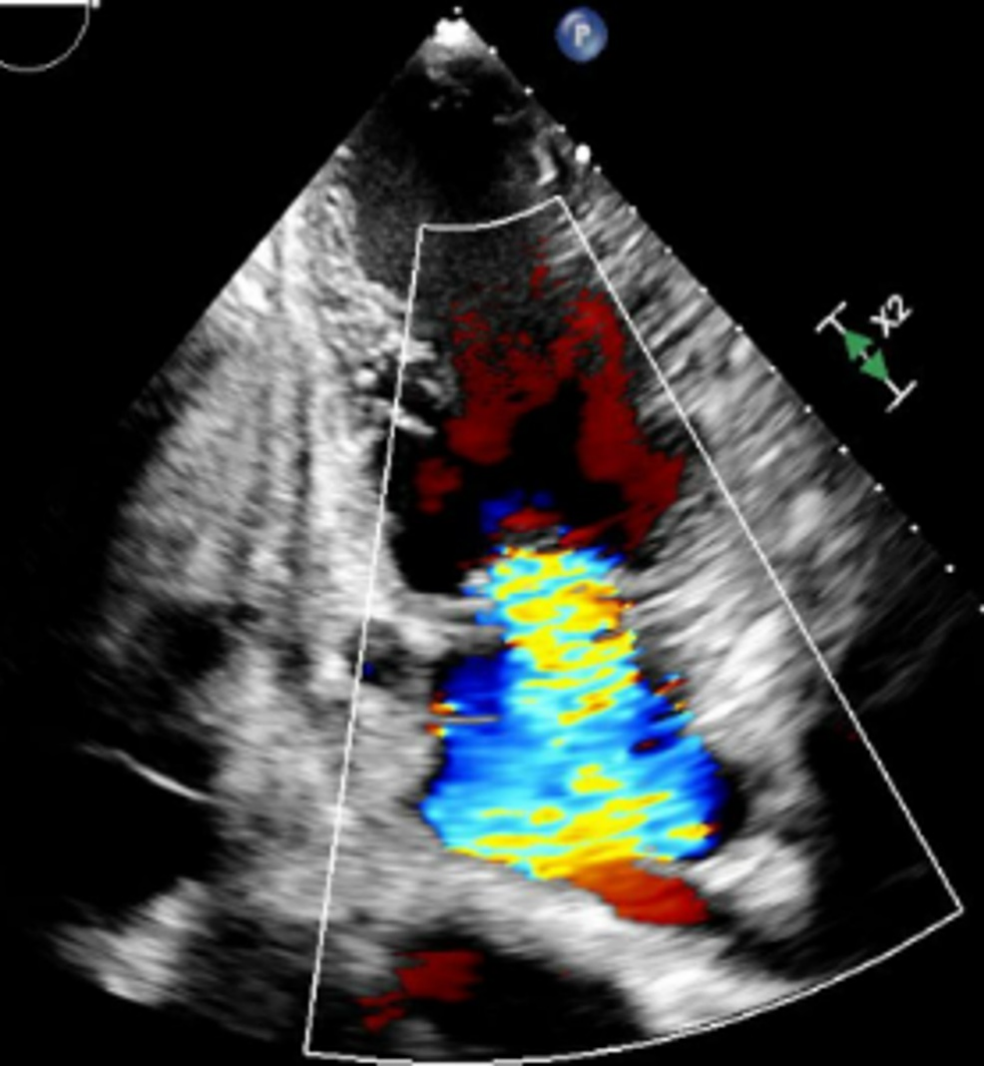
Mitral Regurgitation (two-chamber view on echocardiogram)
